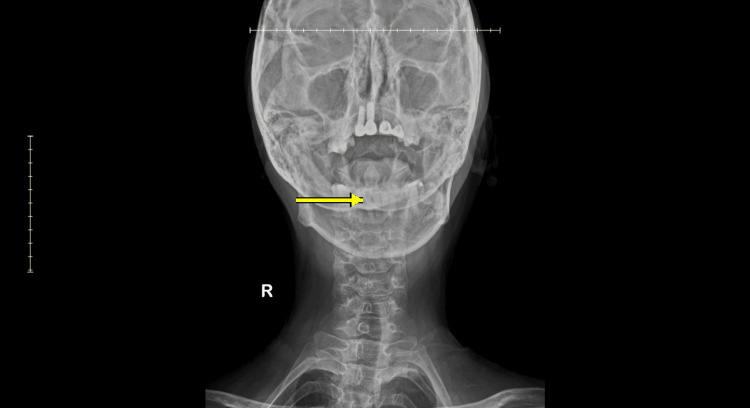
Anteroposterior open-mouth radiograph in neutral position. Arrow indicates the upper cervical region.
